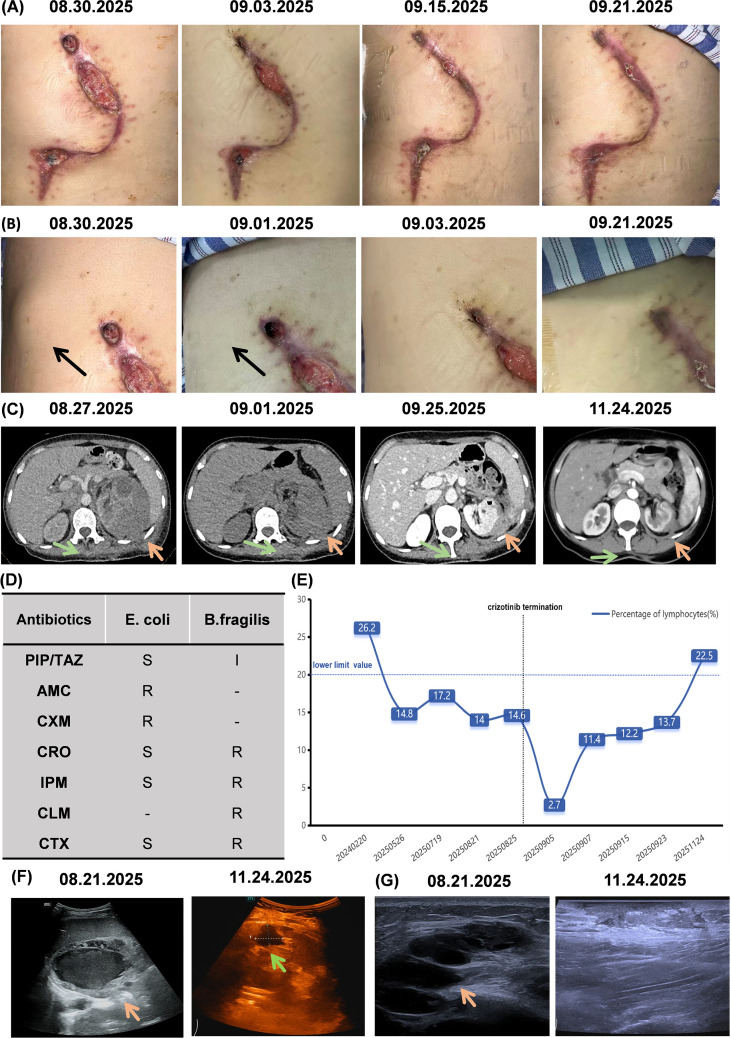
Cases of aseptic inflammatory abscesses of the back and nephritic abscesses following crizotinib-discontinuation and dose-reduction. (A) After discontinuing crizotinib, purulent discharge resolved and the back incision gradually healed. (B) Following crizotinib-discontinuation, the subcutaneous abscess at the 10 o’clock position of the back incision progressively diminished. (C) Abdominal CT: nephritic abscess gradually shrunk after crizotinib-discontinuation, anti-infective therapy and drainage. After the patient began tapering crizotinib, the nephritic abscess continued to shrink as of 2025.11.24; (D) Bacterial culture and sensitivity results from nephritic abscess drainage fluid and blood cultures: Escherichia coli and Bacteroides fragilis infection. Both organisms were susceptible to piperacillin-tazobactam; (E) Lymphocyte Percentage: Prior to the aseptic inflammatory abscesses detection, the patient’s lymphocyte percentage was within normal range. Following abscesses detection, the percentage fell below normal range. After discontinuing crizotinib, the lymphocyte percentage returned to normal range; (F) Color Doppler ultrasound findings: nephritic abscess prior to crizotinib-discontinuation on 2025.08.21; after crizotinib-discontinuation and dose reduction on 2025.11.24, the nephritic abscess showed significant reduction in size compared to previous assessment; (G) Color Doppler ultrasound findings: August 21, 2025 (pre-discontinuation): Aseptic inflammatory abscesses of the back. November 24, 2025 (post-discontinuation, after resuming crizotinib at reduced dosage): Aseptic inflammatory abscesses of the back had resolved. E. coli, Escherichia coli; B. fragili, Fragilis Bacterium; PIP/TAZ, Piperacillin/Tazobactam; AMC, Amoxicillin/Clavulanate; CXM, Cefuroxime; CRO, Ceftriaxone; IPM, Imipenem; CLM, Clindamycin; CTX, Cefotaxime.
